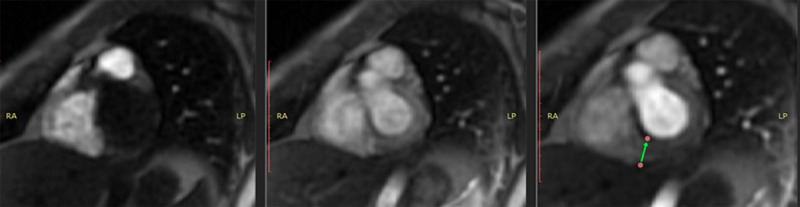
Cardiac MRI showing hyperemia in the basal portion.
